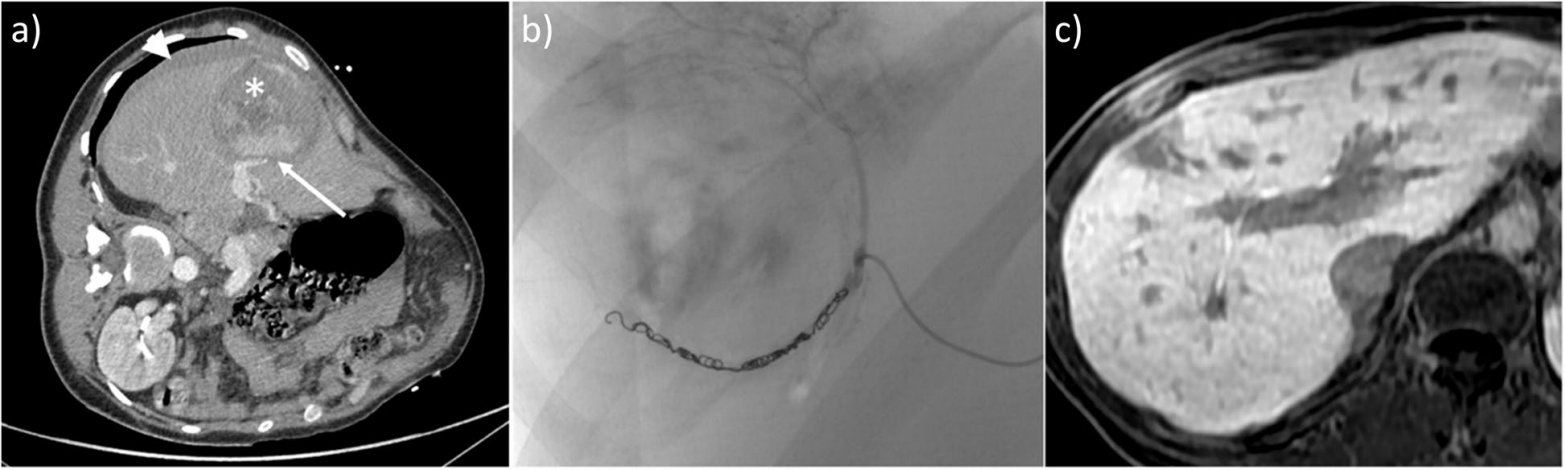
Hepatocellular adenoma evolution during pregnancy in a 29-year-old woman, 39th week of pregnancy, presenting with sudden and strong acute pain in right upper abdomen, associated drop of hemoglobin levels (Hb = 10 g/dL). Axial CT scan on delayed phase (a) shows a tumor (*) measuring more than 5 cm, with active bleeding (arrow) and hemoperitoneum (arrowhead). Due to patient instability, arterial embolization combining gelatin sponge injection and selective distal coiling was performed (b) followed by fetal extraction. Six years after the event, axial scan, T1w MRI (c) shows shrinkage of the lesion
